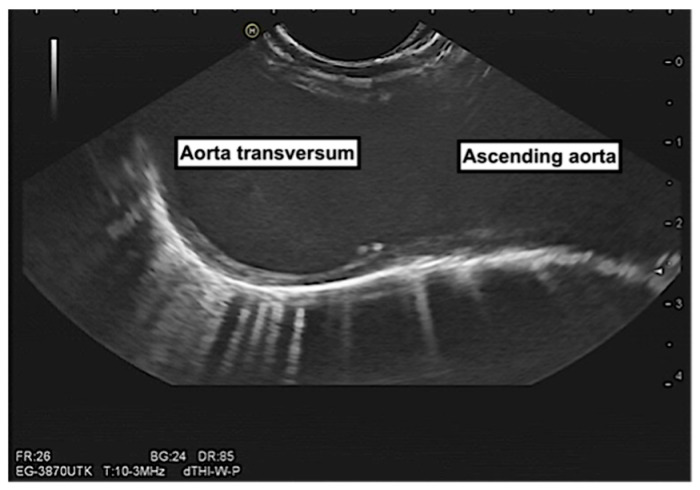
Endoscopic ultrasound demonstrated the anatomy of ascending and transversus aorta, respectively.
